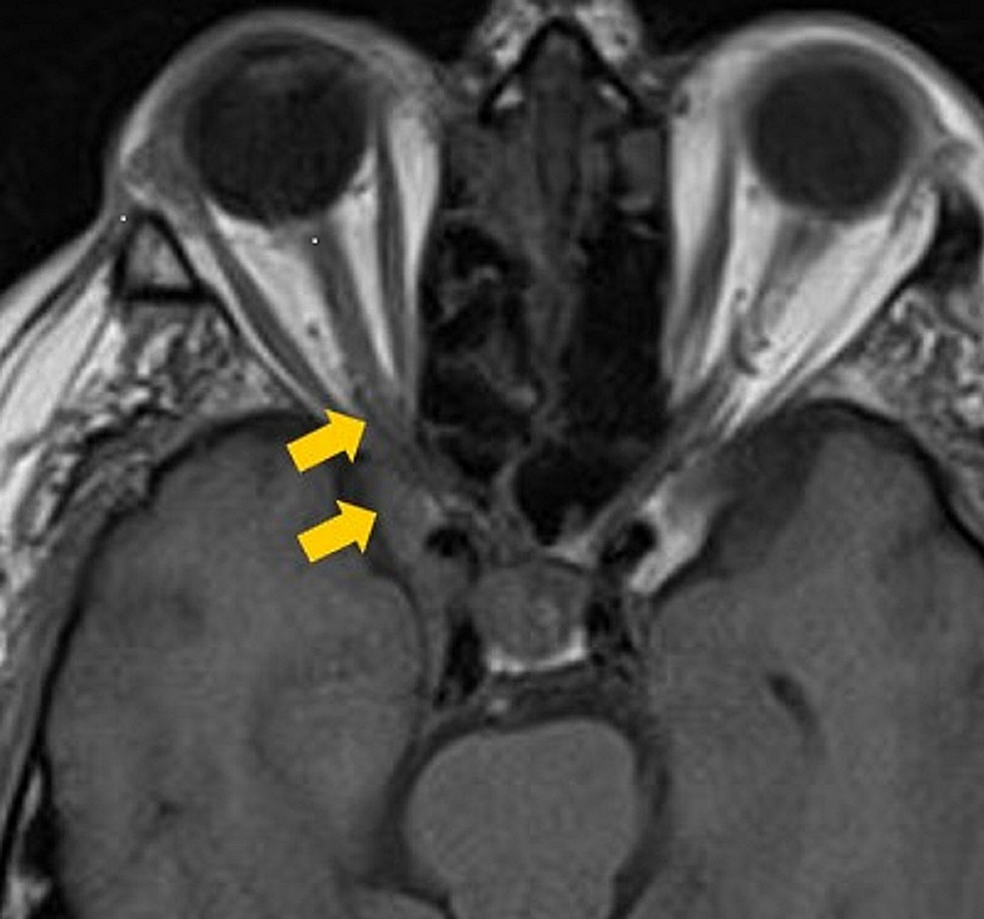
Initial axial pre-contrast T1-weighted MRI The image is demonstrating inflammatory changes in the right posterior orbital apex (yellow arrow) consistent with Tolosa-Hunt syndrome.
